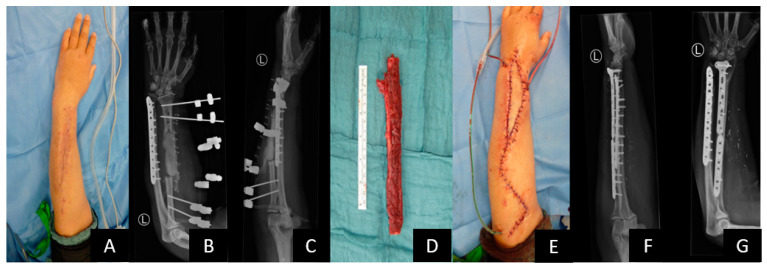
Example of vascularized bone graft. (A–C) Local wound situation and X-ray before reconstruction. Segmental bone defect is filled with a gentamycin spacer and stabilized with external fixation. (D) Free fibula graft prior to inset. (E) Primary closure with the skin island of the osteocutaneous fibula flap. (F,G) Stabile bony union at 12 months after reconstruction.
